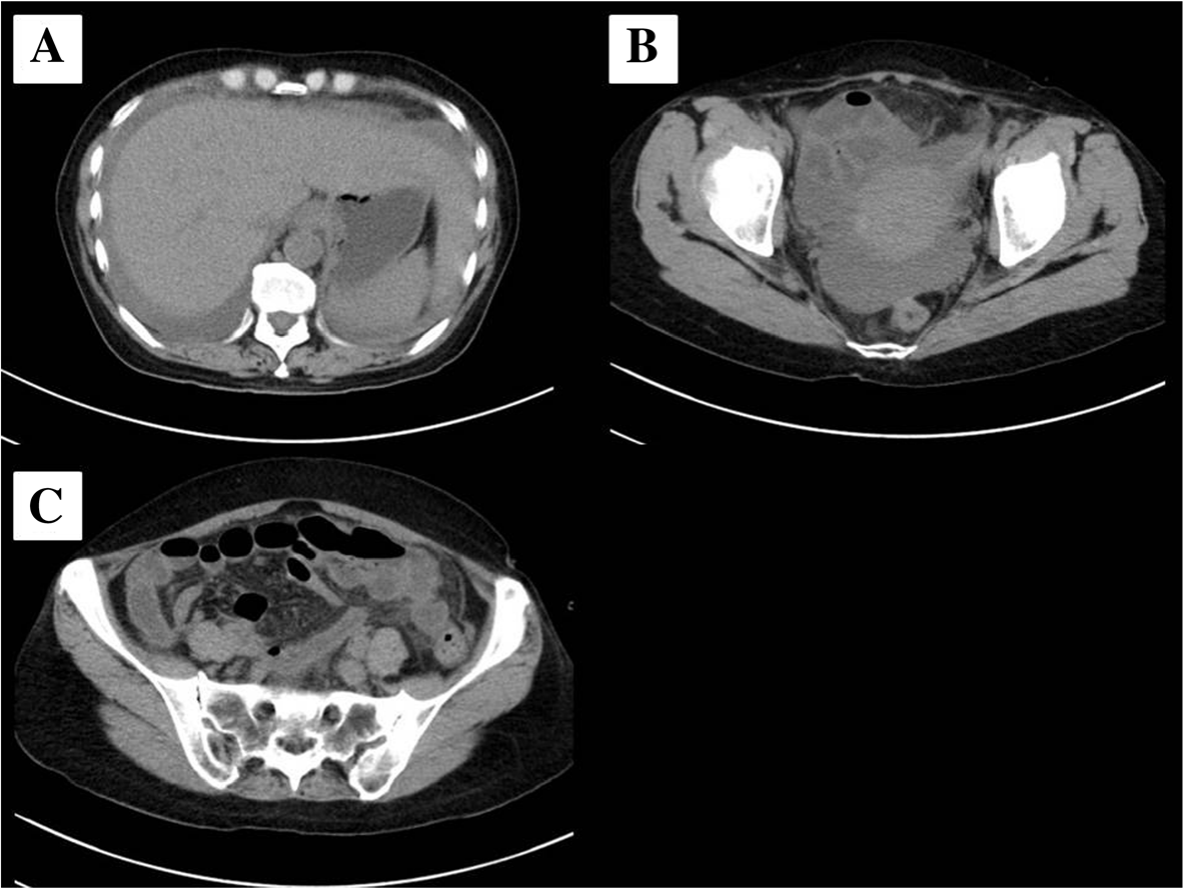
Computed tomography of the abdomen. Computed tomography of the abdomen detected ascites on the liver (a) and Douglas’s fossa (b). CT imaging also showed intestinal edema (c)
